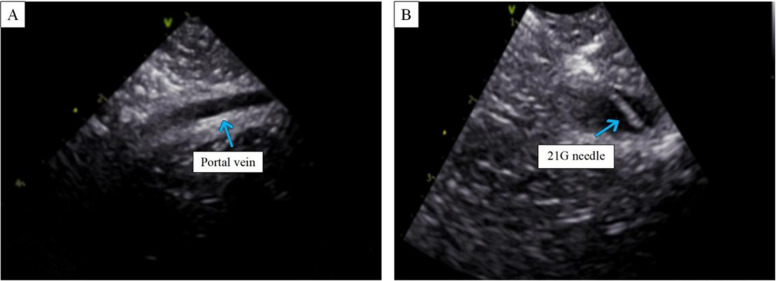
Ultrasound images of the liver and portal vein in vitro. A An observation of the target portal vein using IVNU. B An observation of the 21G puncture needle accessing the target portal vein
